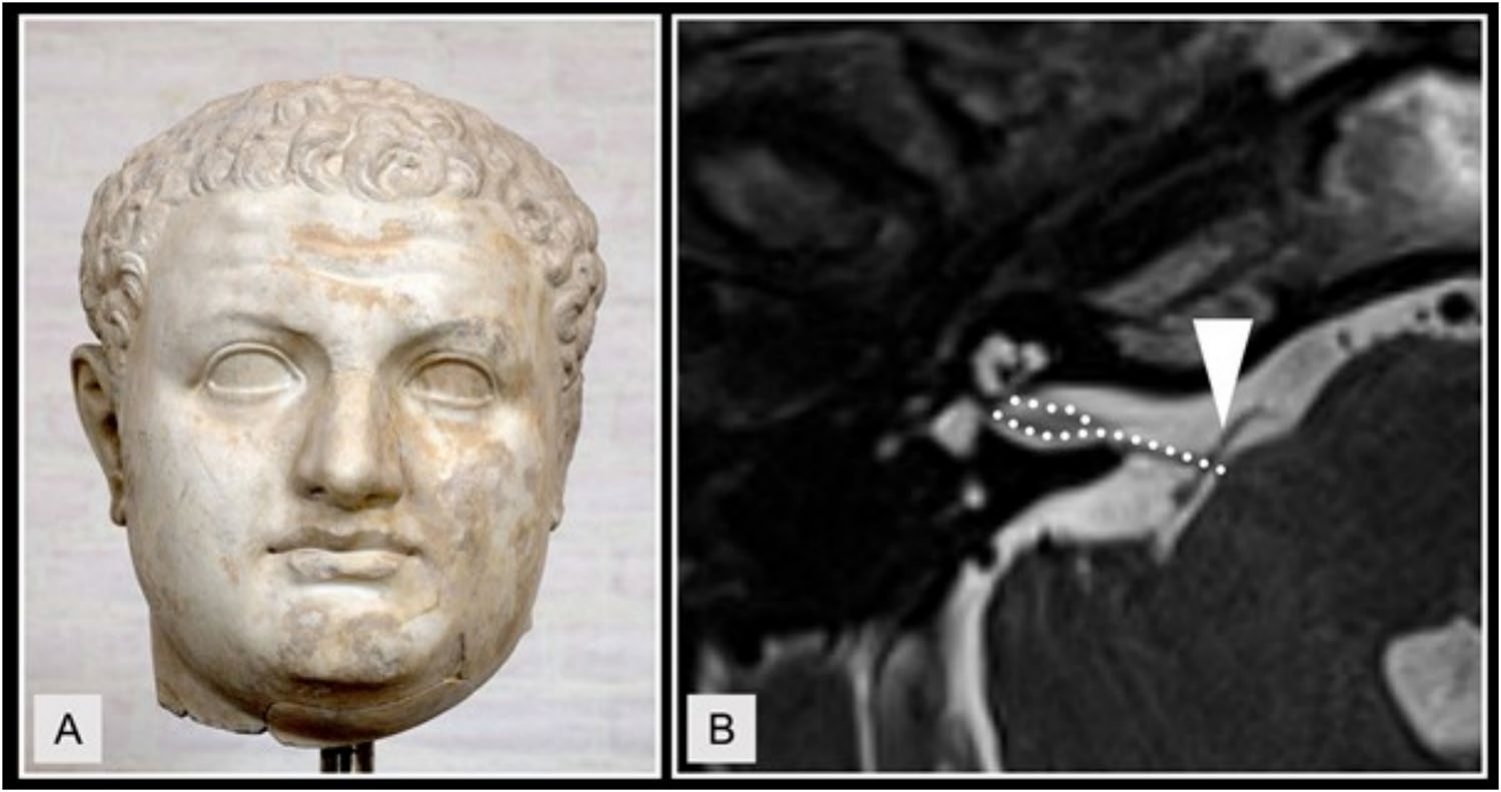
A Bust of the Roman Emperor Titus (39–81 AD), who, according to the Babylonian Talmud, suffered from chronic high-frequency sledgehammer-like sounds in his ear, attributed to a “gnat pecking at his brain”. B Axial section of T2-weighted head MRI, showing a neurovascular conflict (NVC) between the vestibulocochlear nerve (dotted lines) and arterial brainstem vasculature (arrowhead) in a patient with vestibular paroxysmia. Especially NVCs close to the root entry zone of the nerve can cause symptoms such as spells of dizziness and unilateral staccato tinnitus, often referred to as typewriter tinnitus
